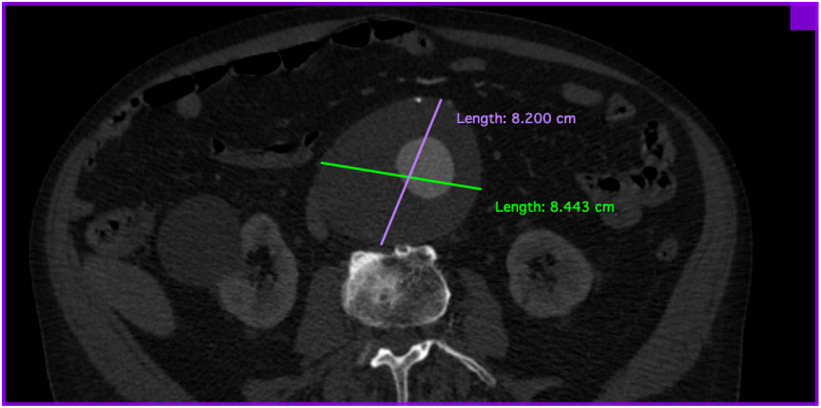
Fig. 1 Preoperative computed tomography image showing a voluminous infrarenal abdominal aortic aneurysm with a transverse diameter of 82×84 mm.
